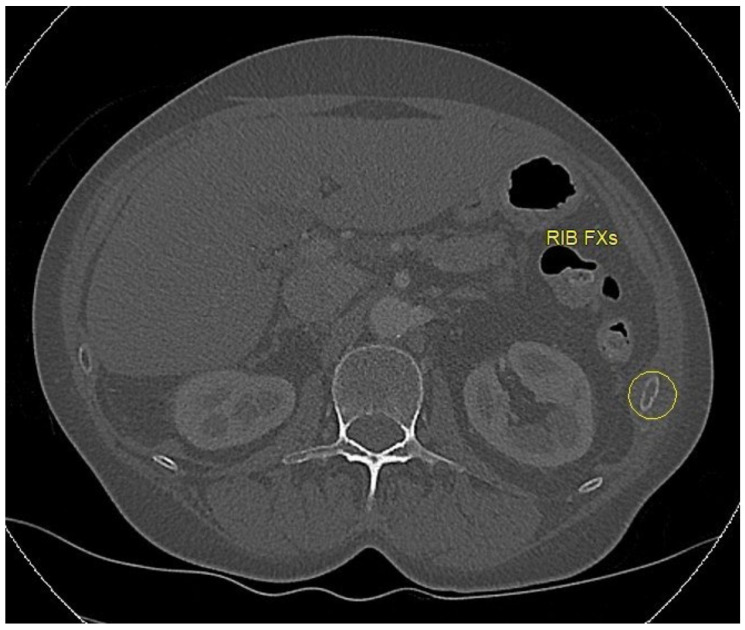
Left rib fracture (circled) as seen on axial CT.
